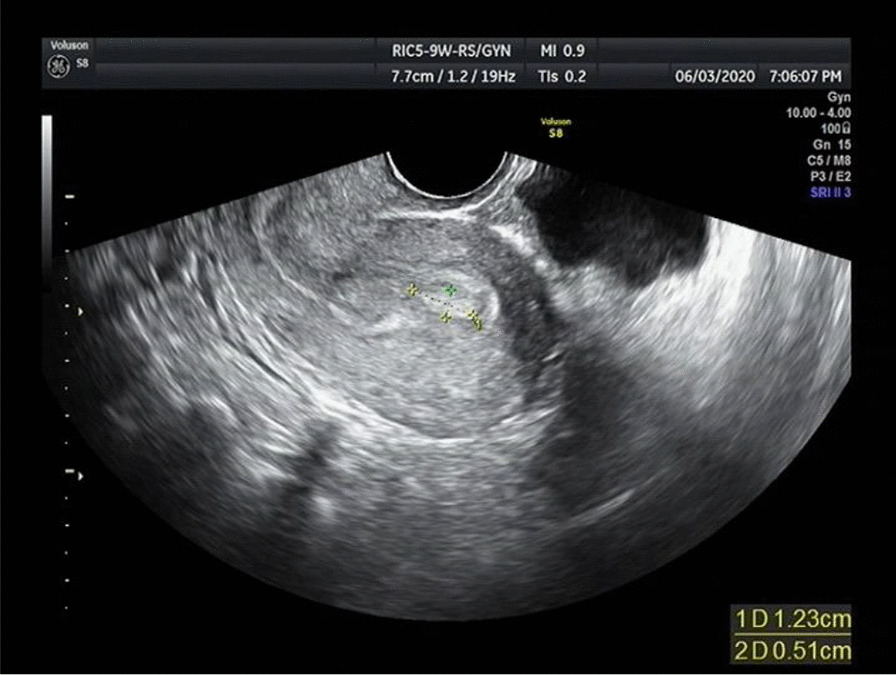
Atypical polypoid adenomyoma on B-ultrasound (endometrium 1.0 cm, intrauterine hyperechoic mass 1.7*1.2 cm)
